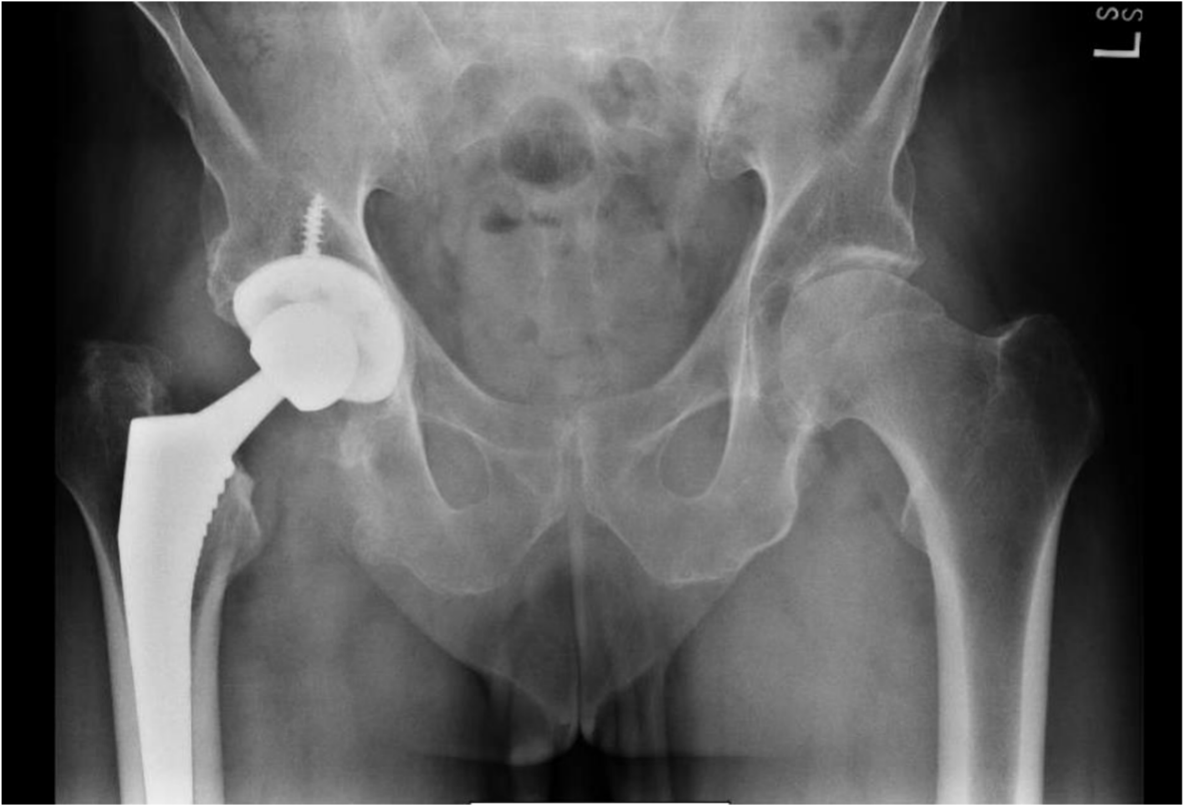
Post-operative radiograph from one of our study participants. For a live surgical demonstration please see the following link: https://www.vumedi.com/video/superpath-the-direct-superior-portal-assisted-total-hip-approach-live-surgery/
